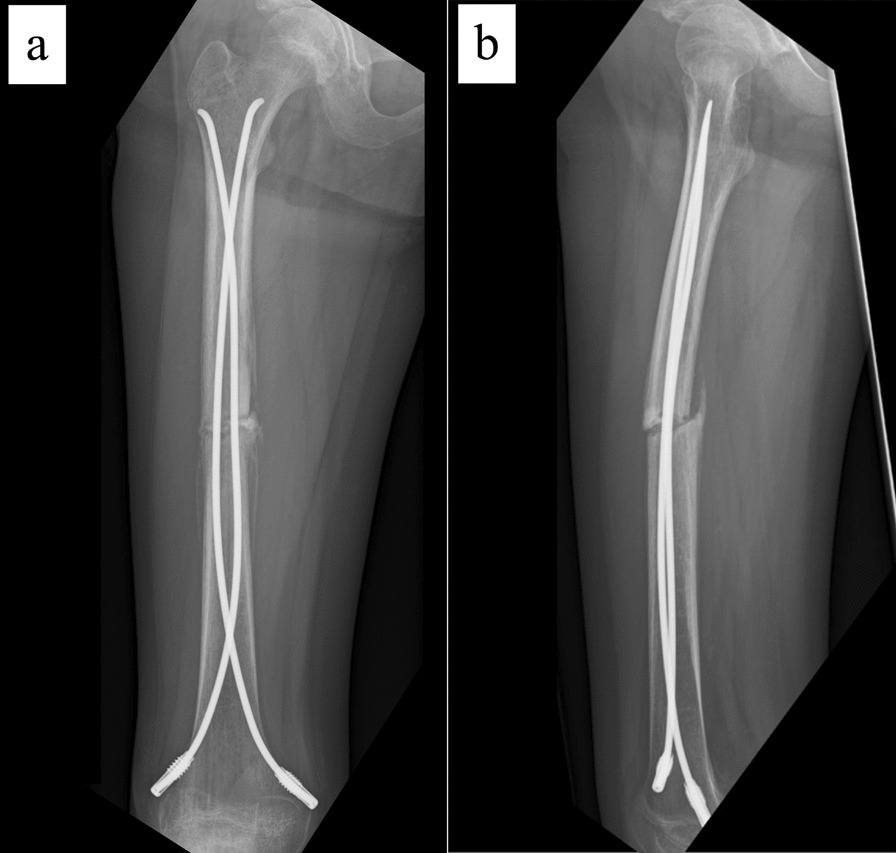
X-ray images at 5 months postoperatively. a Anteroposterior image and b lateral image. Delayed union is observed at the fracture site
